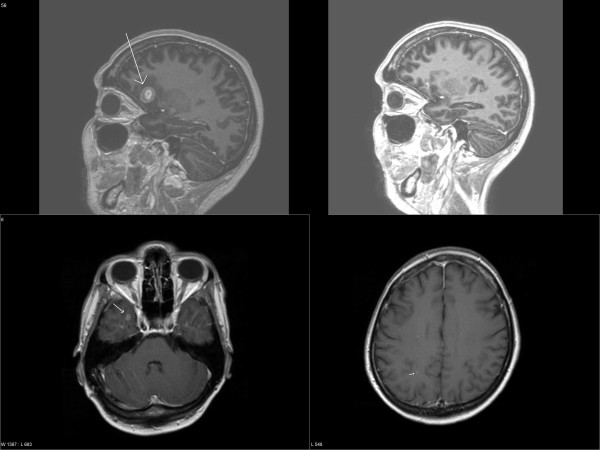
Baseline and follow-up brain imaging. Initial magnetic resonance imaging scans of the brain demonstrating a large frontal mass and multiple small lesions (left side and lower right, August 2006). Small residual frontal abnormality 11 months after whole-brain radiotherapy (upper right).
